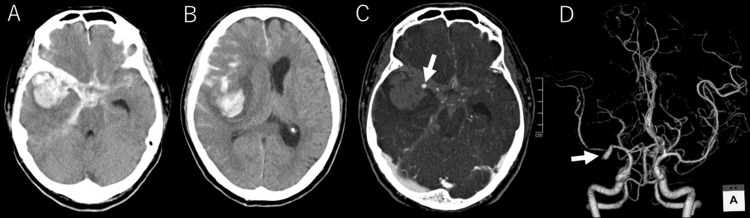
Head CT and CTA. (A, B) Subarachnoid hemorrhage is observed from the basal cistern to the suprasellar cistern, along the bilateral Sylvian fissures, and in the right hemisphere sulci. A subcortical hemorrhage is noted in the right temporal lobe. There is significant compression of the right lateral ventricle with a midline shift to the left. (C, D) A 12 x 3 mm contrast-enhancing mass is observed protruding dorsally from the proximal M1 segment of the right middle cerebral artery, consistent with a pseudoaneurysm (arrow). CTA, computed tomography angiography; CT, computed tomography
